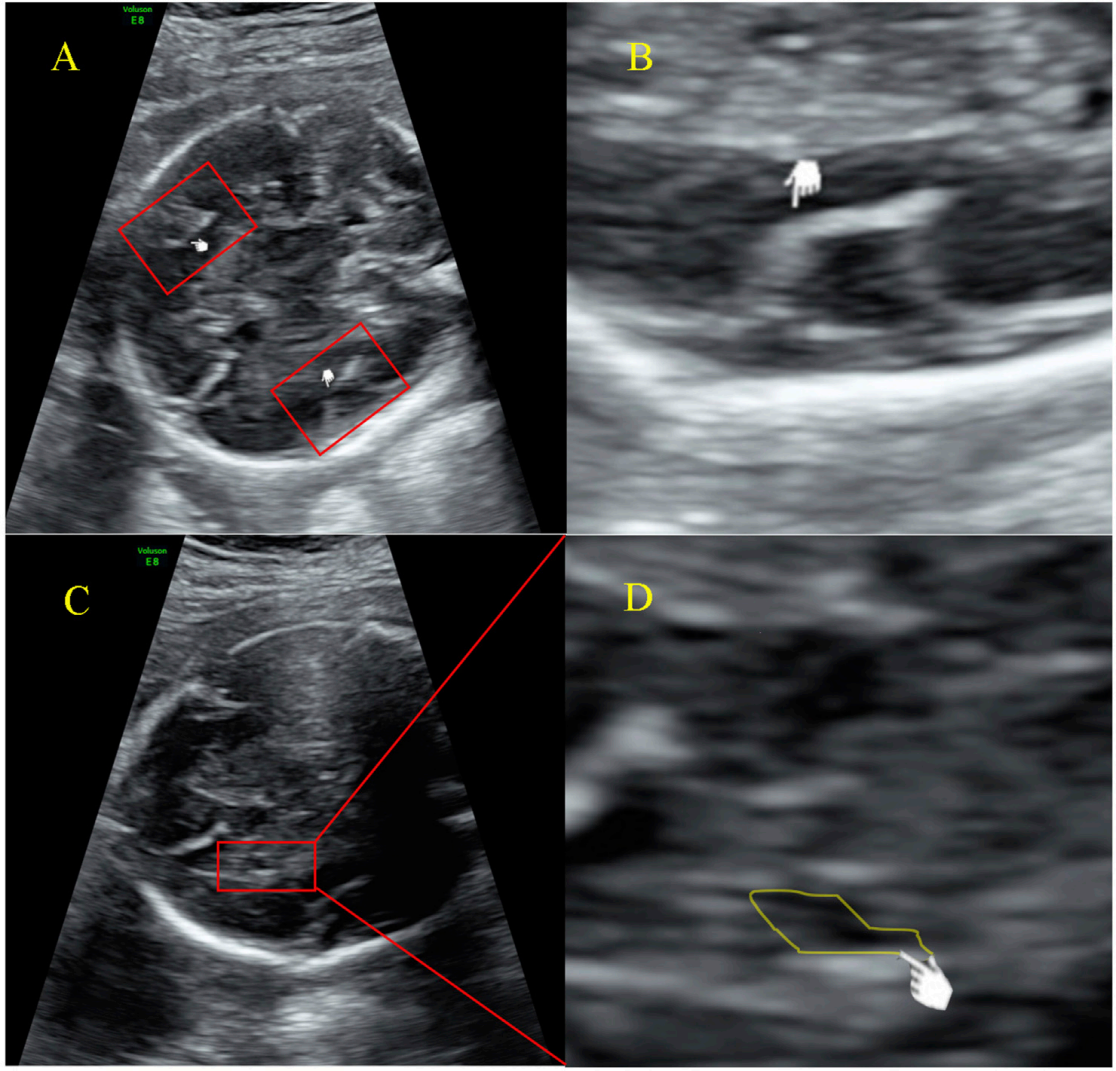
Ultrasound examination image of the fetal (A) Malformation of cortical development indicated by an increased Sylvian fissure angle; (B) Measurement of the Sylvian fissure angle; (C) Subependymal cyst; (D) Magnified image of the indicated cystic area, presented by ultrasonic examination at 25+5 weeks of gestation.
